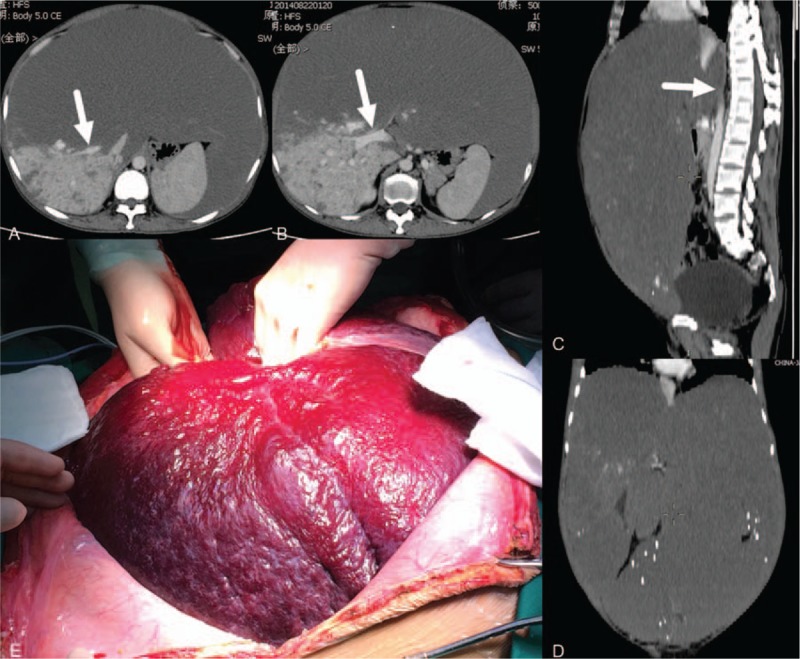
A patient with Kasabach-Merritt syndrome associated with an extremely giant liver hemangioma (50 cm). (A–D) CT images showing an extremely giant liver hemangioma, occupying the entire abdominal cavity and extending to the pelvis, with compression of the right hepatic vein (A, white arrow), right portal vein (B, white arrow), and inferior vena cava (C, white arrow); (E) intraoperative exploration revealed an extremely giant liver hemangioma occupying the entire abdominal cavity.
